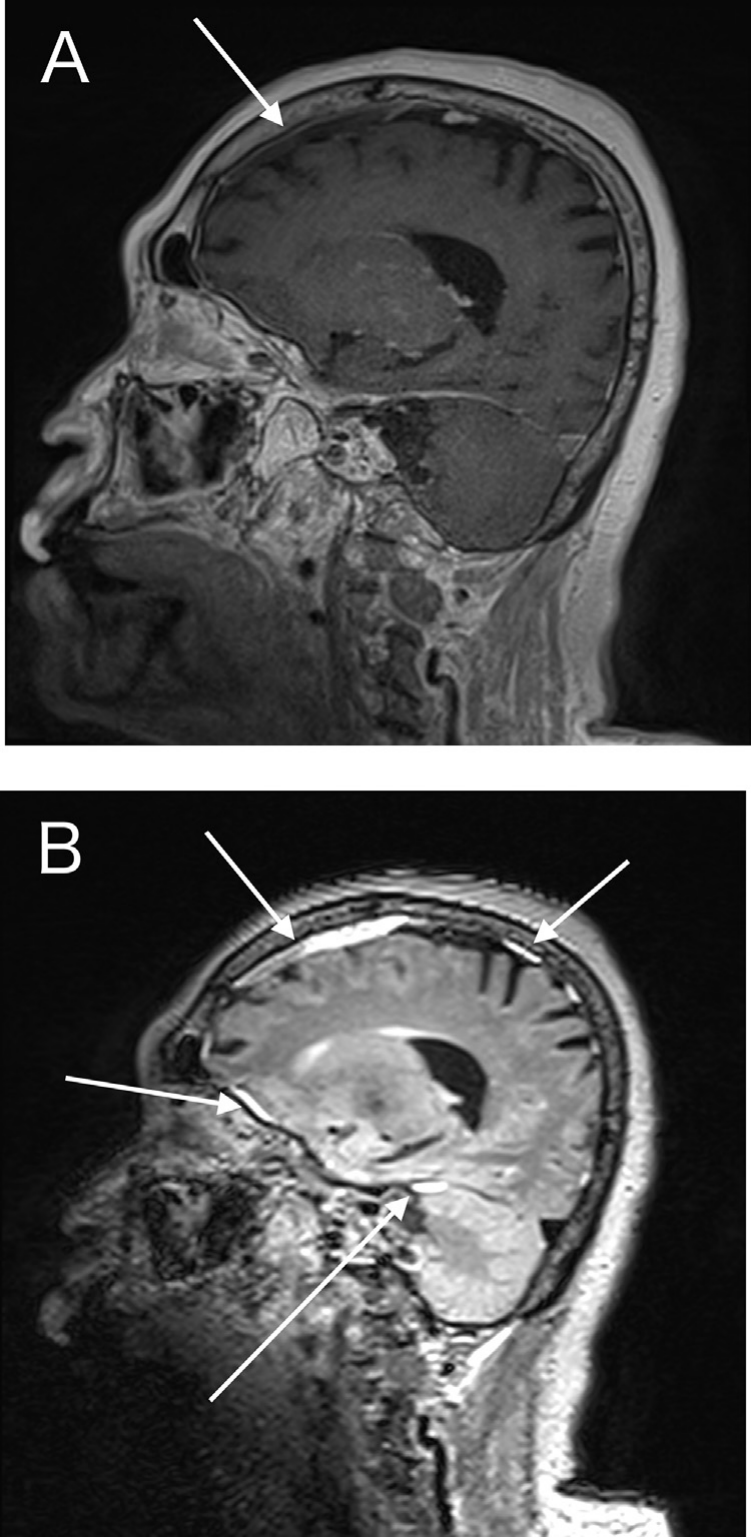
(A) CE-T1WI demonstrated a peripheral linear enhancement of the thickened falx (arrow). (B) The thickened falx was peripherally and partially homogeneously enhanced on the CE-FLAIR images (arrows). The homogeneous enhancement of the falx may reflect high sensitivity to low Gd concentrations in CE-FLAIR.
